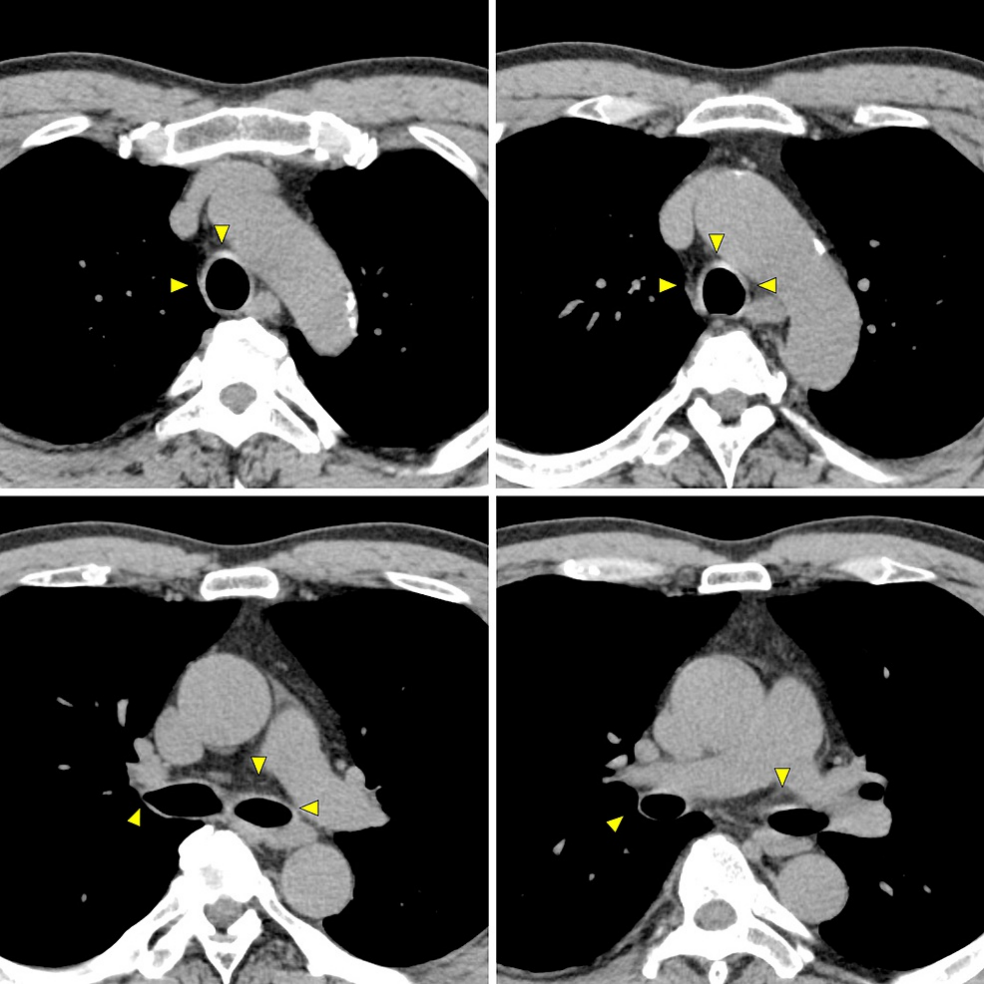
Follow-up chest CT images of the patient after treatment initiation. Improvement was found in the thickened tracheal and bronchial walls (arrows) and in the attenuation of soft tissue around the trachea.
